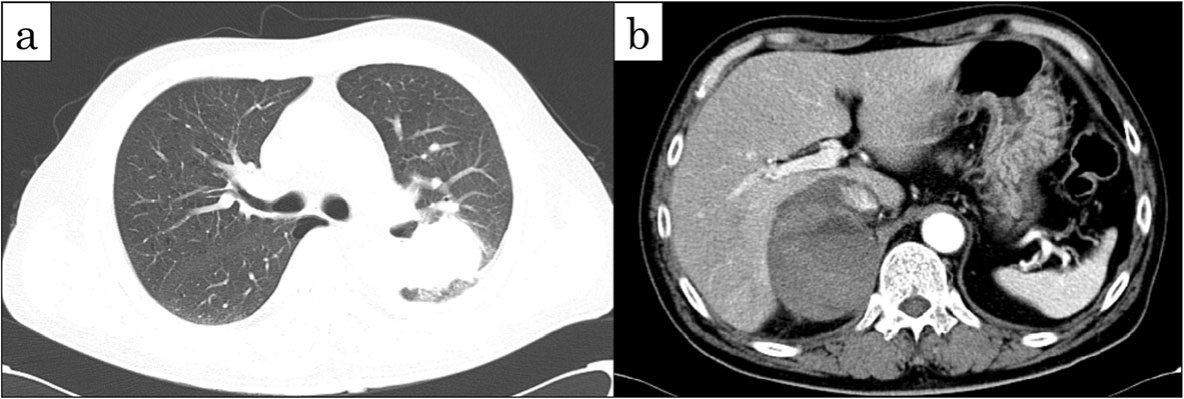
CT scan showed abnormal masses. a Chest CT scan showed a 42-mm tumor in the left lung S1 + 2 progressing to S6. b Abdominal enhanced CT scan showed a 82-mm mass with no active bleeding
